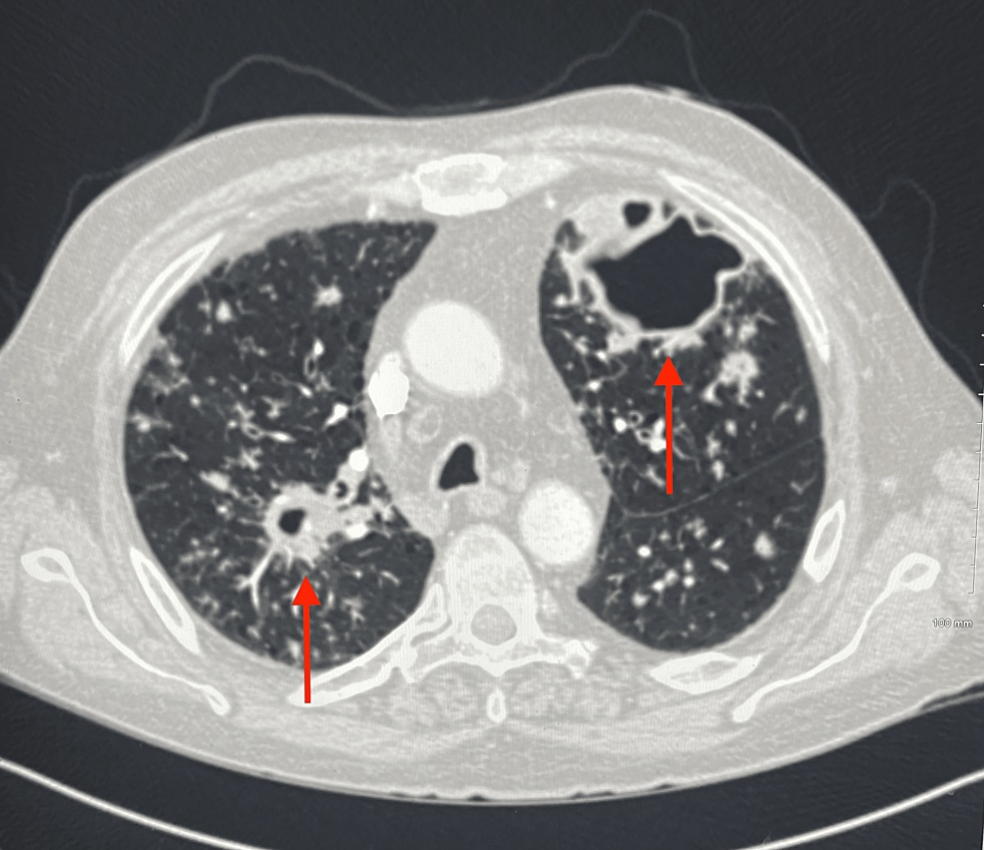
CTA of the chest showing progressive bilateral cavitary disease.
